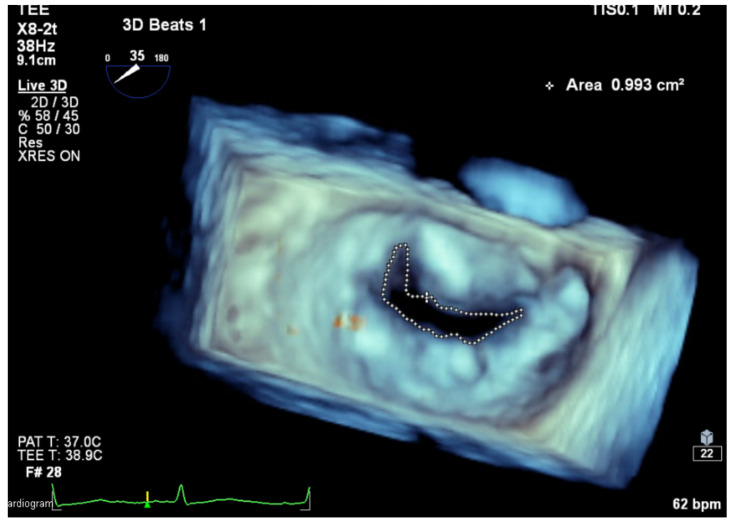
MVA measurement using 3D TEE planimetry. (MVA: mitral valve area, 3D TEE: three-dimensional transesophageal echocardiography).
